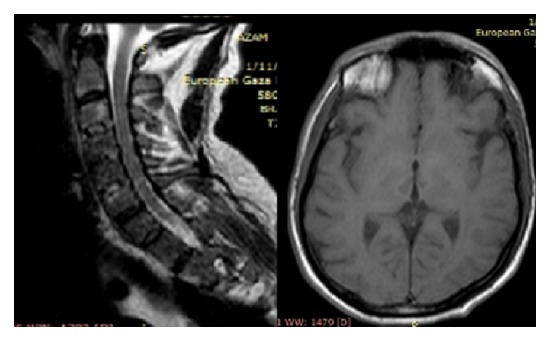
Brain and cervical MRI (after 2 weeks) show bilateral thalamic infarction with no compression on the spinal cord.
